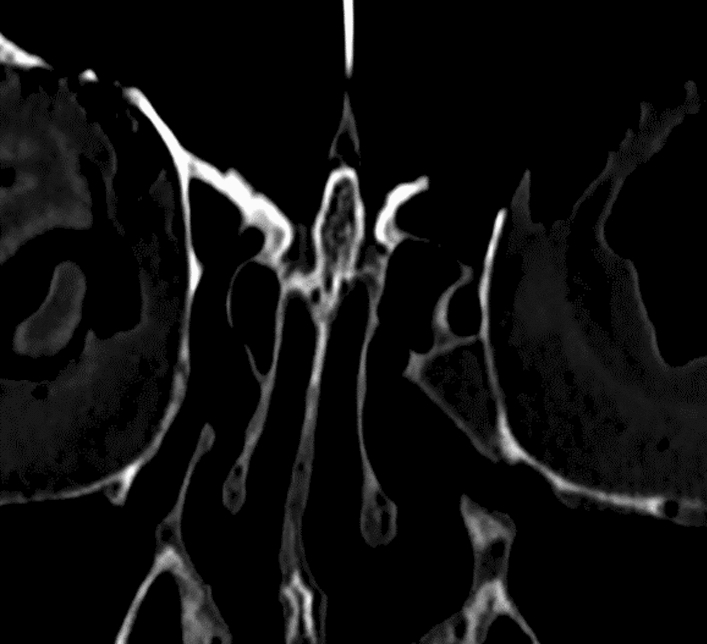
Coronal view CT scan of Crista Galli and Cribriform Plate in Bone Window mode using SECTRA (Linköping, Sweden) IDS7 Software
